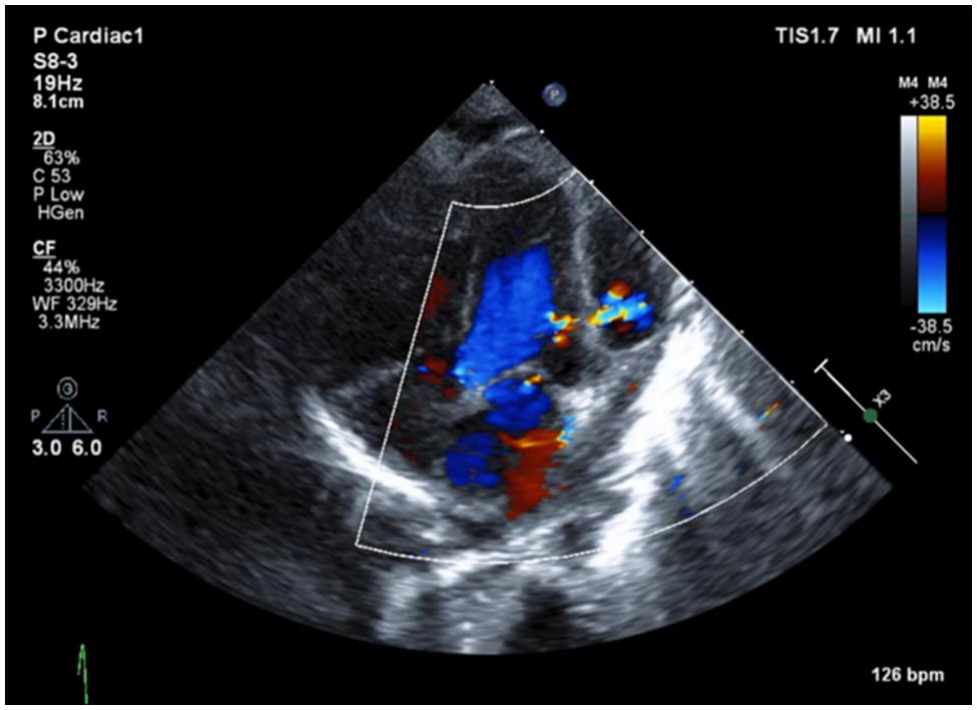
A Cavity is Observed on the Anterior Surface of the Left Ventricle, and Colour Doppler Imaging Reveals Blood Flow Entering from the Left Ventricle.
